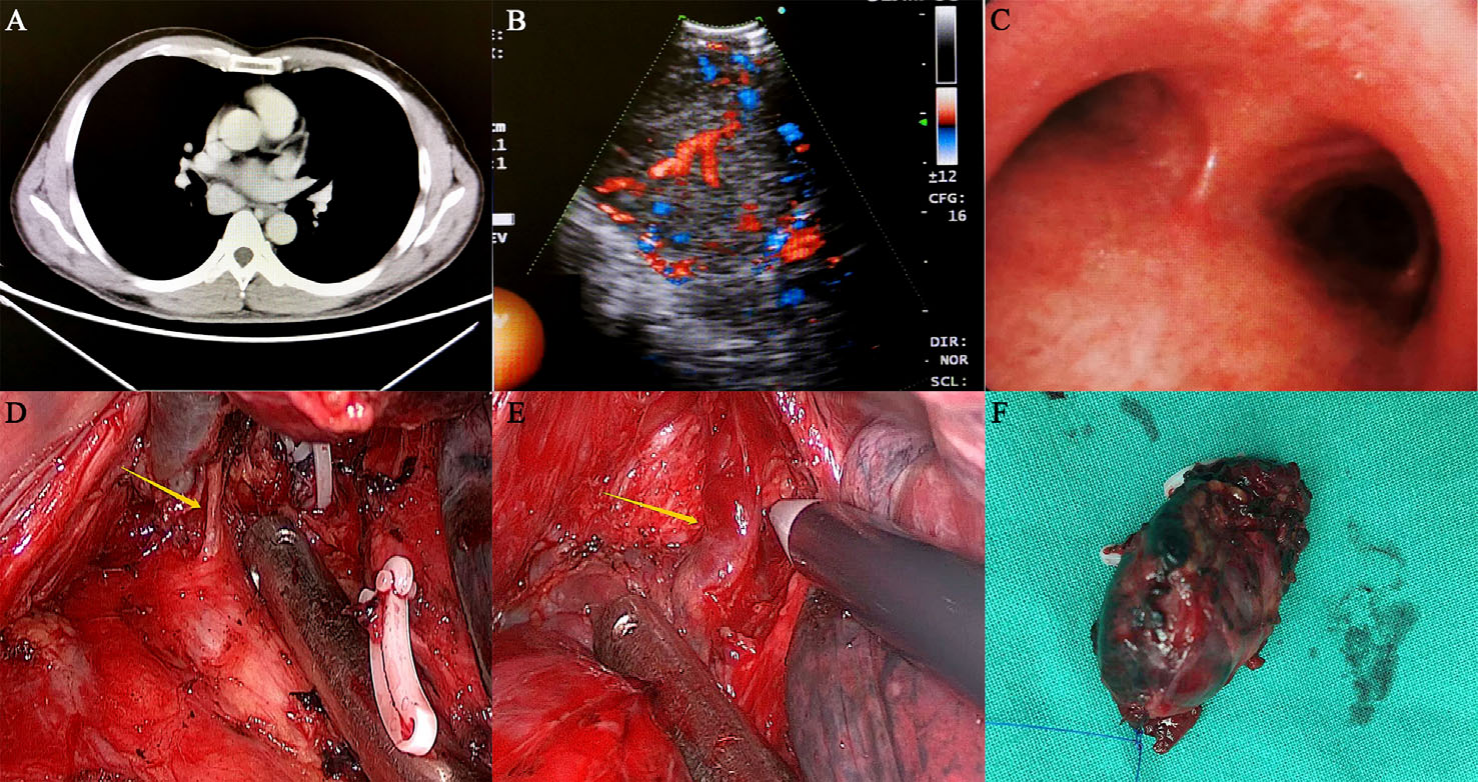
Preoperative examination and intraoperative status of the patient. (A) The thoracic contrast-enhanced CT showed that the mass was located in the right posterior mediastinum, and its size was approximately 40*35 mm. The CT value of the plain scan was approximately 46 HU, and the CT value was approximately 130 HU after enhancement. (B, C) Endobronchial ultrasonography showed that a posterior mediastinal mass was located far from the carina with a complete capsule, clear margin and adequate blood supply. (D, E) During the operation, the lesion was located under the carina and surrounded by several abnormally large bronchial arteries, which had an extremely abundant blood supply. (F) The mass was completely removed and was approximately 3.5 cm in size.
